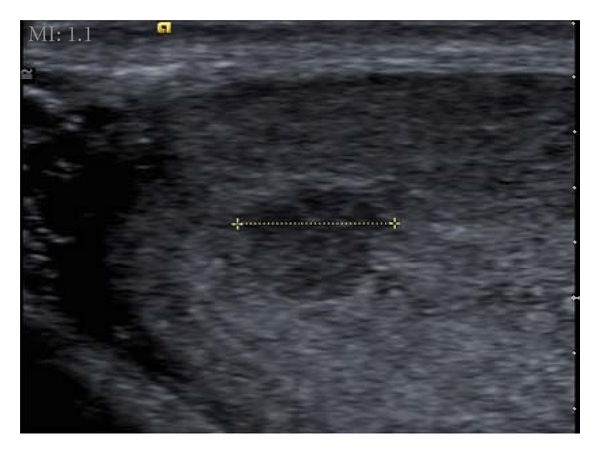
Gray-scale ultrasound image reveals a hypoechoic solid mass within the left testis.
